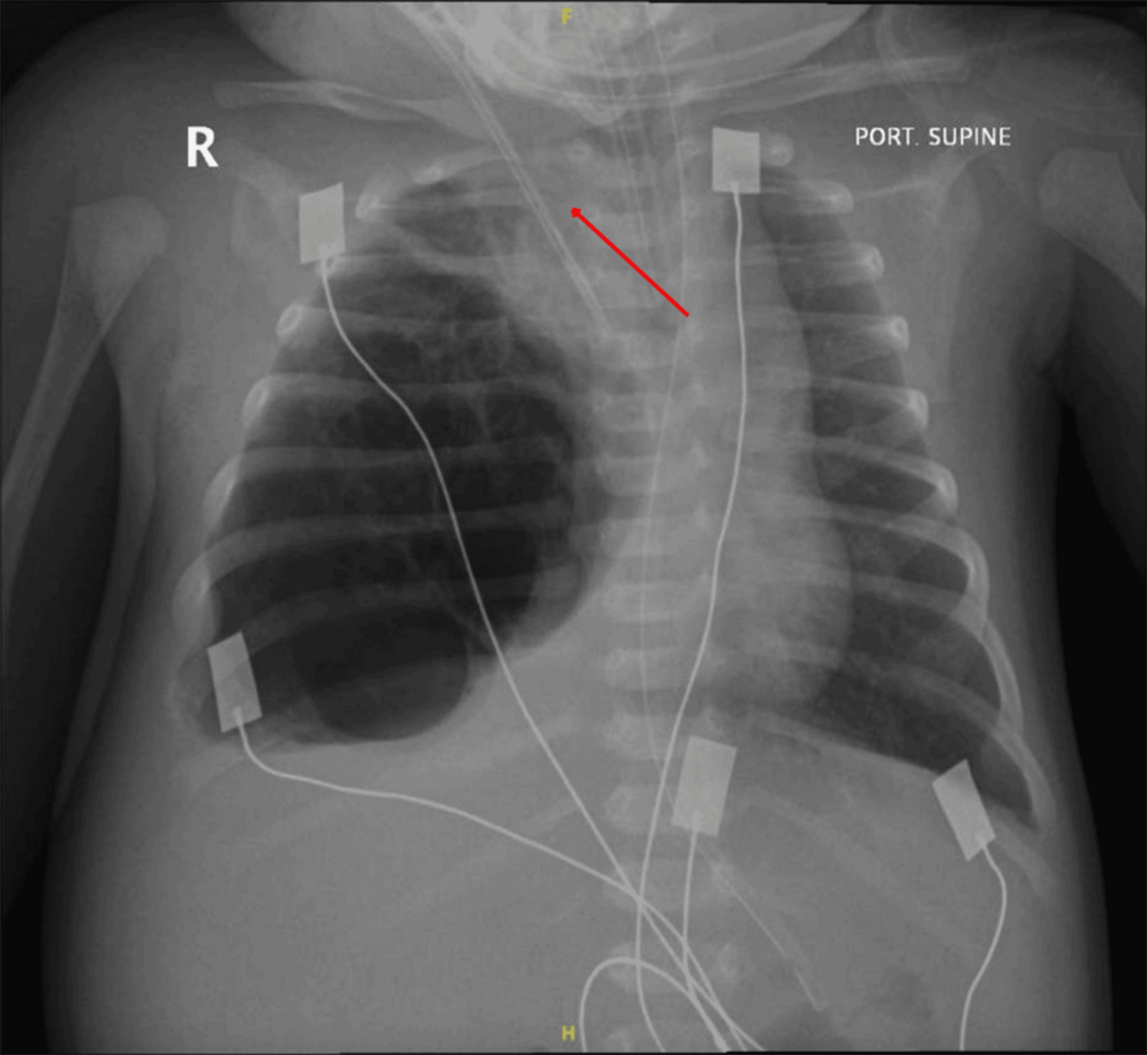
Preoperative anteroposterior chest radiograph at four weeks of age The red arrow indicates a significant mediastinal shift caused by overinflation of the right hemithorax, secondary to the macrocystic CPAM lesion. CPAM, congenital pulmonary airway malformation
